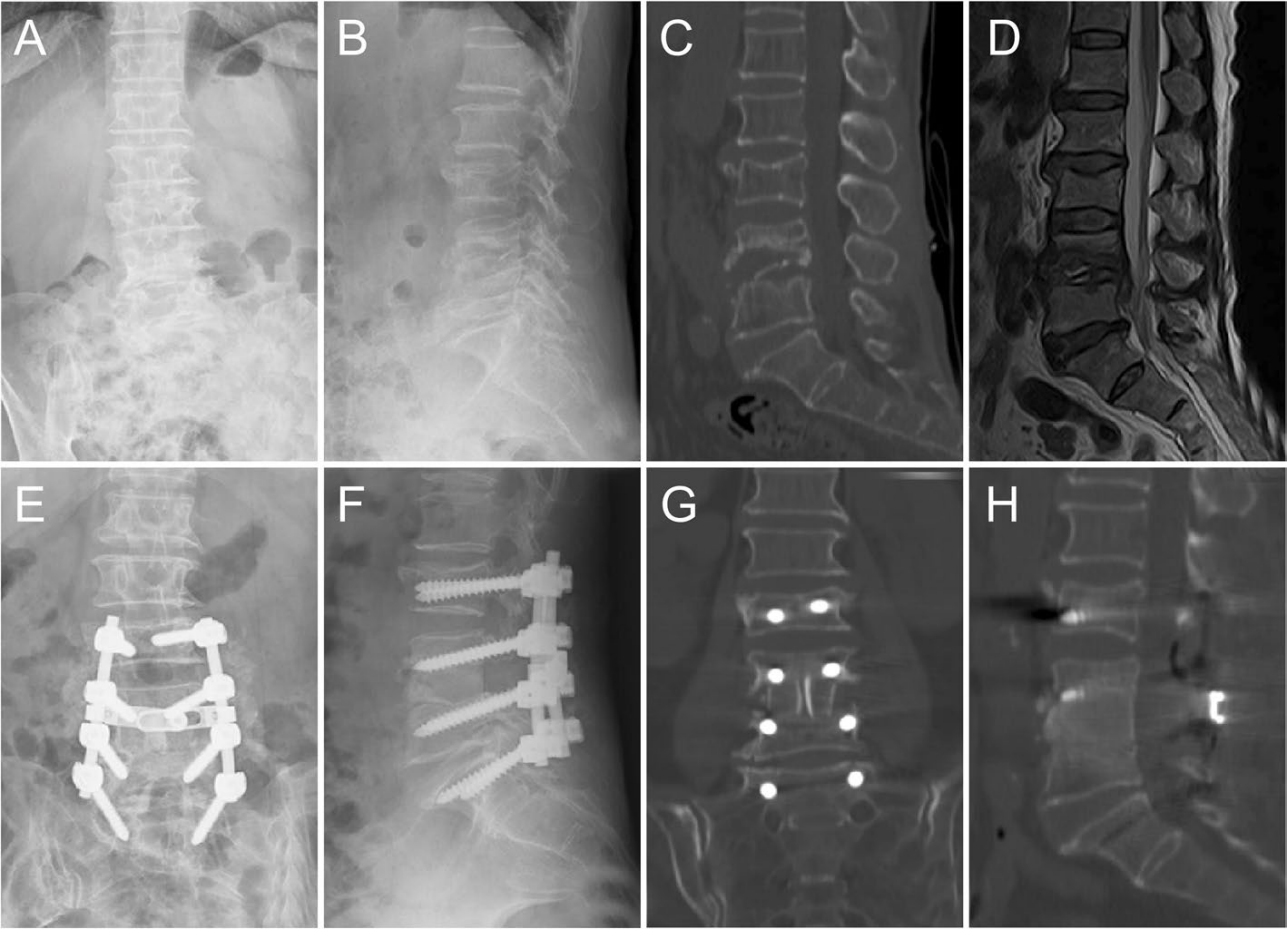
An old male with destructive L4-L5 was treated by posterior bilateral laminectomy debridement and bone grafting fusion combined with internal fixation. Figures 3A, B, C, and D are preoperative radiological images showing destructive bone at the L4 -5. Two years after the operation, the X-ray images (E, F) and CT images (G, H) show solid bone fusion
